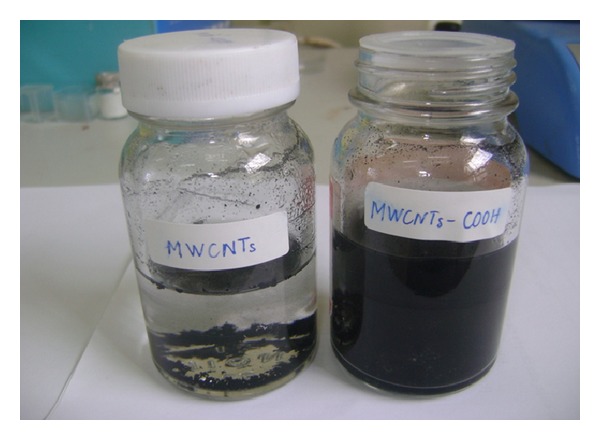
The typical image of MWCNTs-COOH was well dispersed in water after 30-minute ultrasonic vibration.
